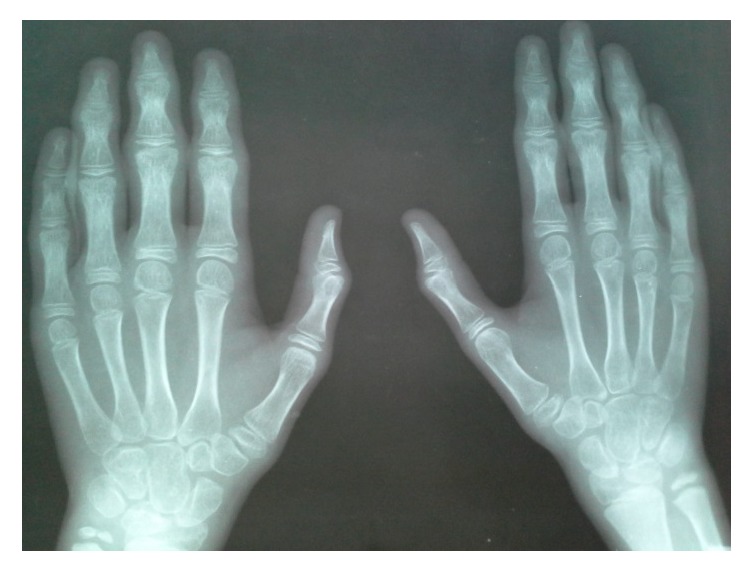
X-ray of hands showing epiphyseal enlargement of the metacarpophalangeal and interphalangeal joints with juxta-articular osteopenia.
